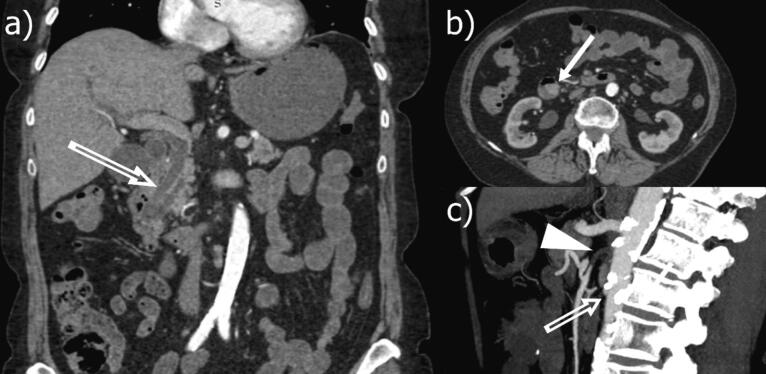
Preoperative CT-reconstruction. a) Double-duct sign shows dilation of bile duct and pancreatic duct (blank arrow). b) Tumor at the ampulla of Vater (filled arrow). c) Total occlusion of the superior mesenteric artery (arrowhead); atherosclerotic plaques at the abdominal aorta (unfilled arrow).
